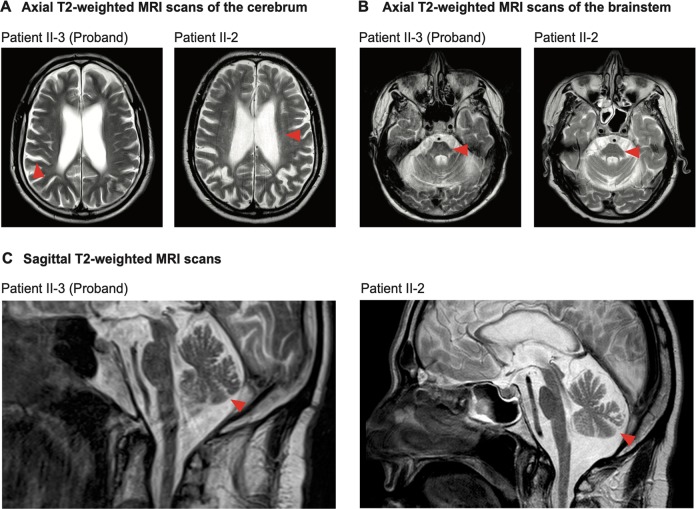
Axial and sagittal T2-weighted MRI scans of the patients’ brains. a Axial T2-weighted MRI scans of the patients’ cerebrum. Red arrows denote areas of extensive atrophy. For patient II-2 the red arrow also denotes small, discrete, periventricular T2-hyperintense white matter lesions. b Axial T2-weighted MRI scans of the patients’ brainstem. Red arrows denote atrophy in the pons. c Sagittal T2-weighted MRI scans of the patients. Red arrows denote atrophy in the cerebellum.
